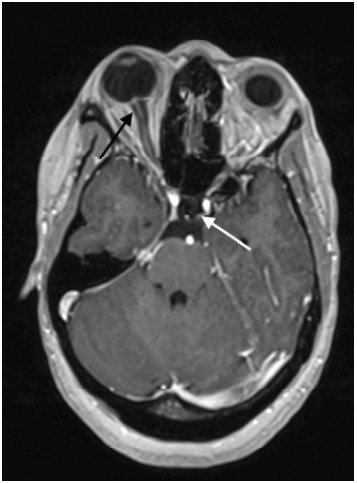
Axial T1 gadolinium enhanced magnetic resonance image of patient’s brain. Patient was found to have multiple findings consistent with idiopathic intracranial hypertension including partially empty sella (white arrow) and prominent optic nerve sheath and papilledema (black arrow).
